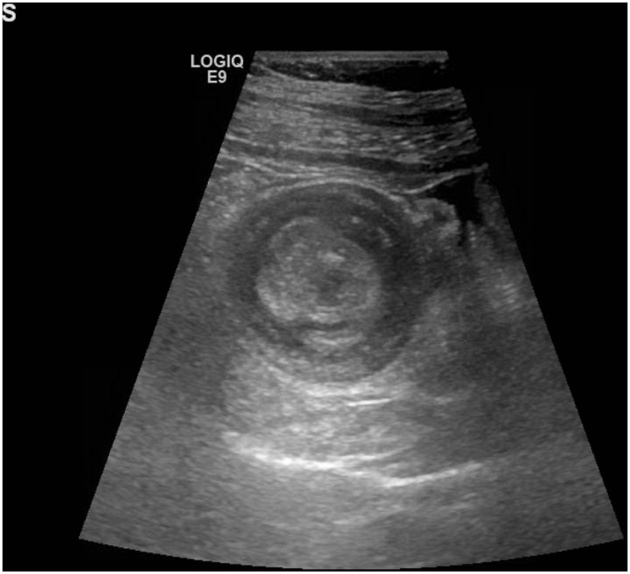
Abdominal ultrasound, transverse view, revealed doughnut or bull s’ sign, a pattern of intussusception. The patient gave his written consent to report this case and his clinical images.
